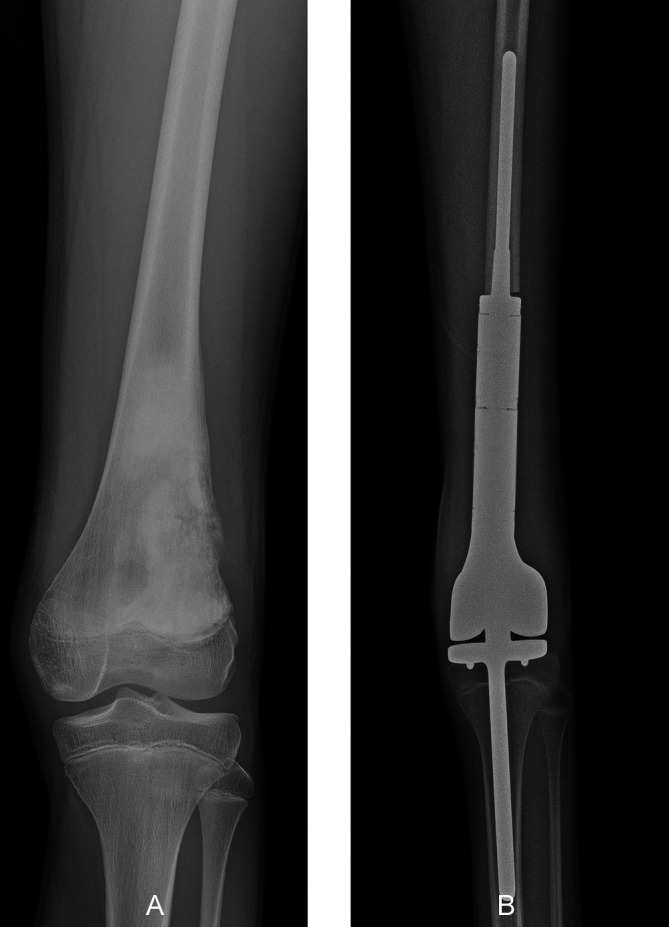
A 12-year-old boy diagnosed with osteosarcoma of the left distal femur, who was treated by osteoarticular resection followed by modular prosthesis reconstruction. (A) Preoperative anteroposterior radiographic view of the knee showing a permeating sclerotic lesion of the distal femur. (B) An immediate postoperative radiograph showing reconstruction of the distal femur using a modular prosthesis with a smooth tibial stem. The modular distal femur prosthesis with smooth tibial stem was able to preserve the growth of the growth plate of the proximal tibia in children with sarcoma of the distal femur.
